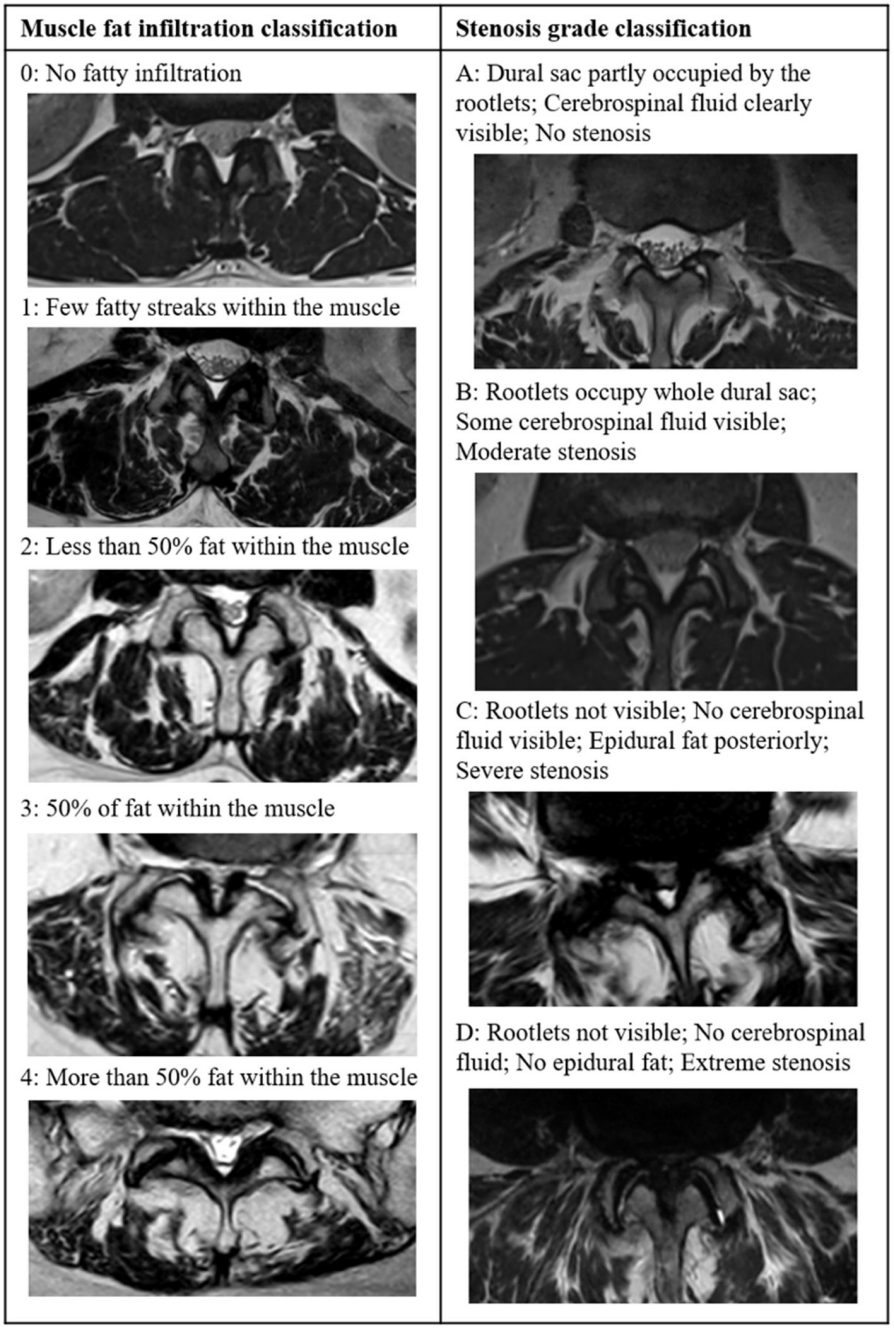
T2-weighted axial images showing the grading of fatty infiltration of paraspinal muscles according to the Goutallier classification (left) (27) and grading of lumbar spinal stenosis according to the Schizas classifications (right) (36).
